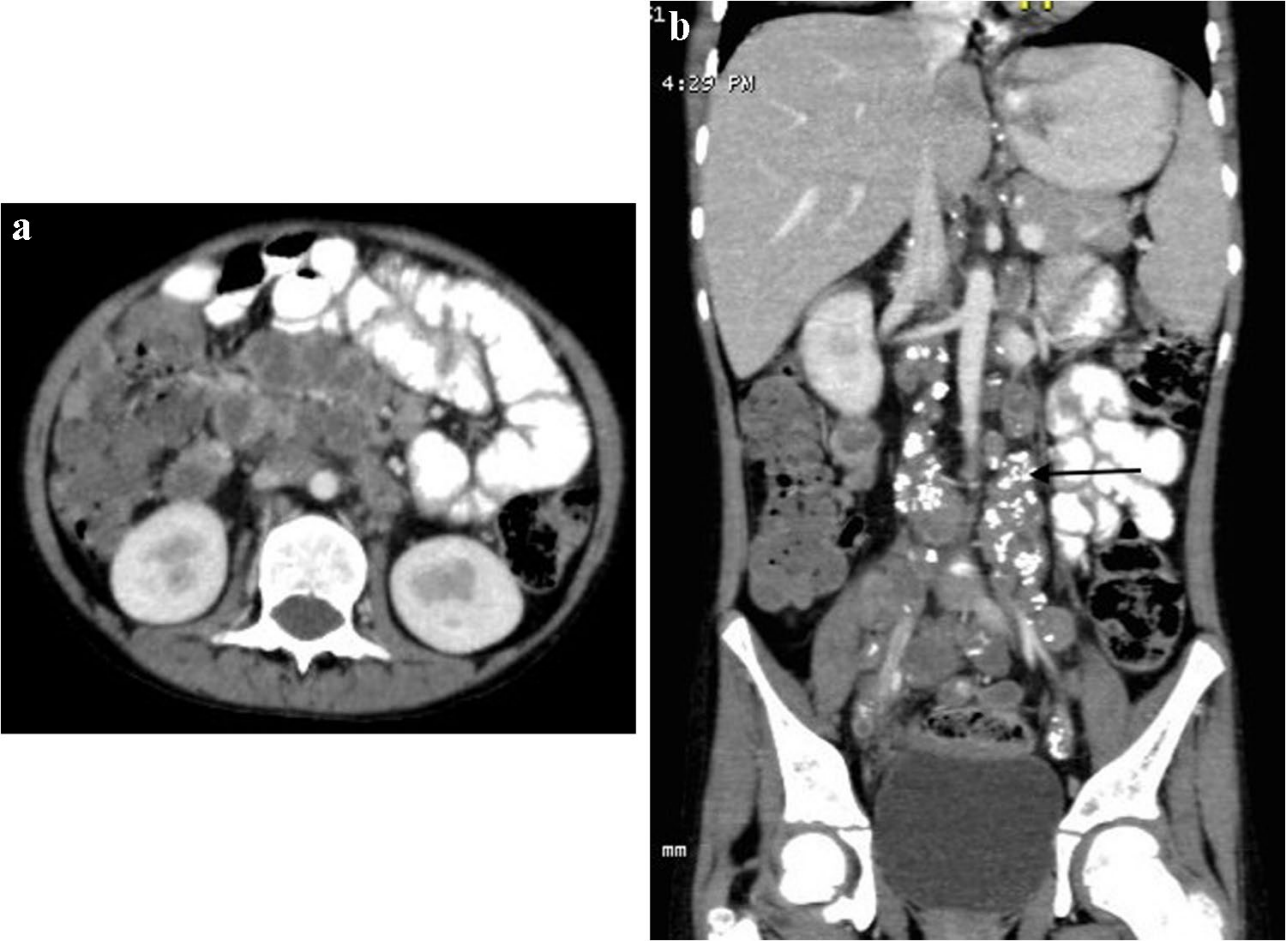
A 9-year-old boy with ileocecal tuberculosis. Axial (a) and coronal (b) contrast-enhanced computed tomography images show circumferential mural thickening involving the caecum and terminal ileum. Enlarged calcified lymph nodes are also seen on the coronal image (*arrow*)
